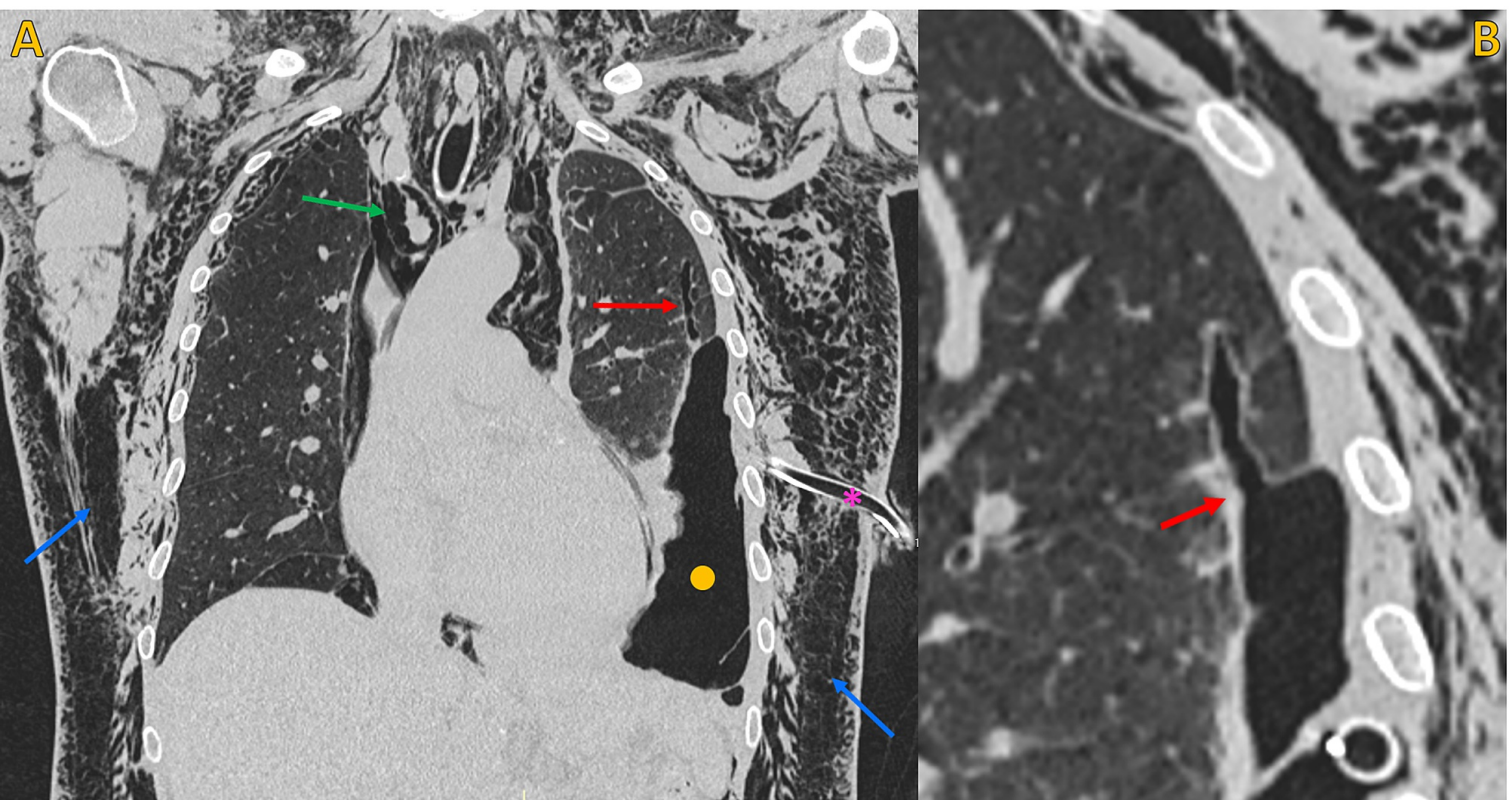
Non-contrast chest CT, lung window Non-contrast chest CT, lung window. A) Coronal plane and B) Oblique coronal plane (magnified view), shows the point of communication between a peripheral enlarged bronchiolar structure and the pleural space, compatible with a bronchopleural fistula (red arrow). Note extensive soft tissue emphysema (blue arrows), large pneumomediastinum (green arrow) and left pneumothorax (yellow circle). Chest tube (pink asterisk).
